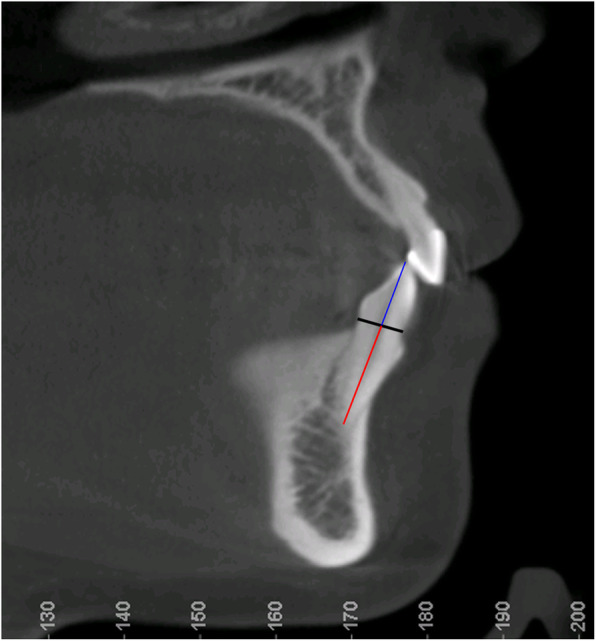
Measurement of tooth crown length (blue line) and root length (red line) on CBCT
